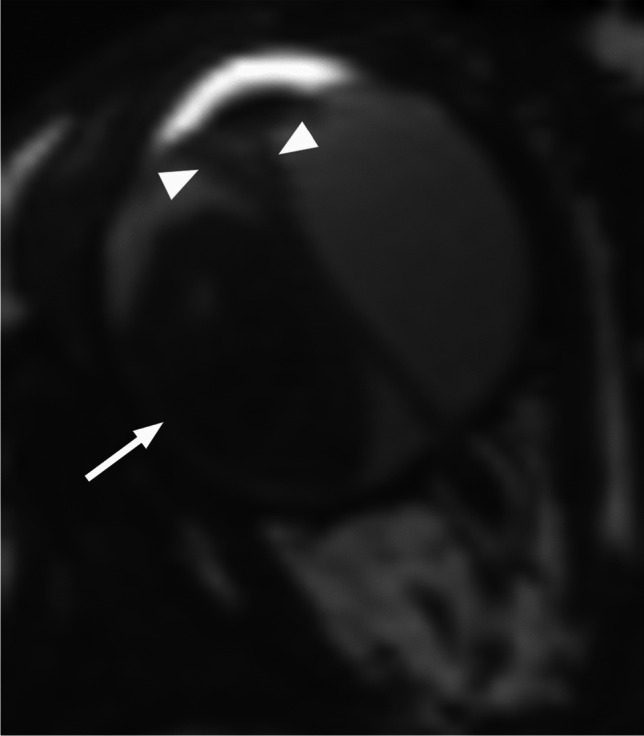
Exophytic growth of retinoblastoma (arrow) with a prominent V-shaped retinal detachment (arrowheads) on axial T2-weighted image through the globe
